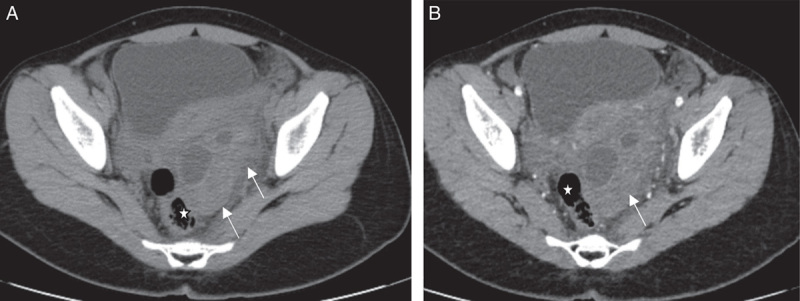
(A) Non-contrast computed tomography (CT) image shows a heterogeneous lesion with cystic spaces (white arrows) in the left adnexa, causing a mass effect to the rectum (white star). (B) Contrast-enhanced computed tomography image shows heterogeneously enhancing lesions with some cystic spaces (white arrow). This lesion can be confused with the enlarged ovary with peripherally placed follicles. Rectum is shown by a white star.
